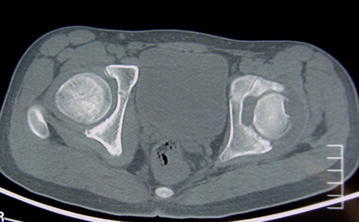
CT images of bilateral hips. The left femoral head was irregular with rough margins, the bone trabeculae were unclear; cortical bone fracture and small free bone fragments were observed
